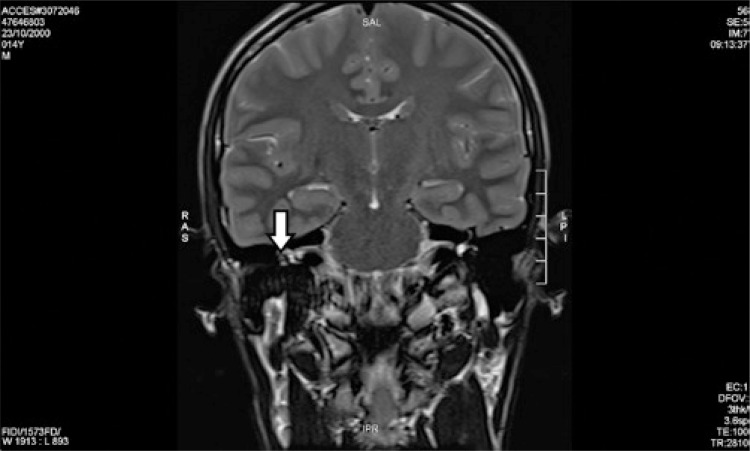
Coronal T2-weighted cranial magnetic resonance imaging view showing hemangioma (white arrow) of the right facial nerve
